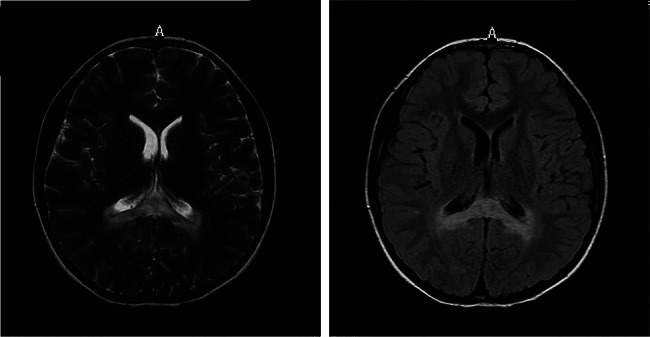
Brain MRI (patient 1): The long T2 signal intensity (left) and FLAIR image high signal intensity (right) on the splenium of the corpus callosum and the posterior side of the adjacent bilateral ventricles
